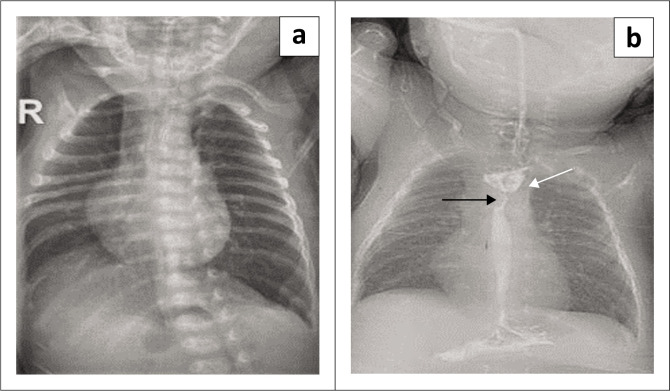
Postoperative tracheo-oesophageal fistula complication. The child presented with difficulty in feeding and tolerating a semisolid diet. (a) The chest radiograph revealed hyperinflated lungs with flattened diaphragmatic domes. (b) Contrast was administered via the mouth, revealing a stricture in the mid-thoracic oesophagus (black arrow) and a dilated proximal oesophagus (white arrow).
